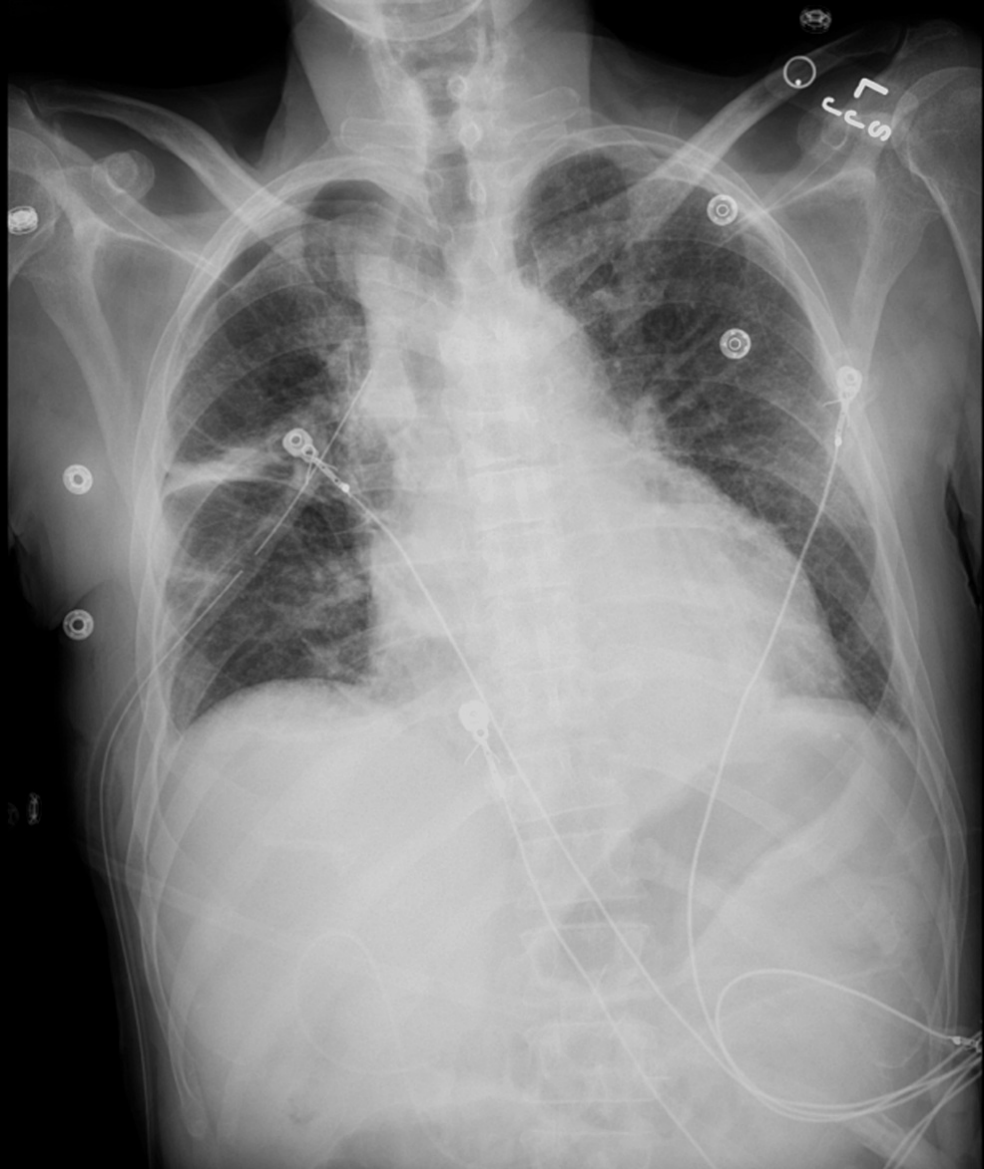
Chest X-ray post right-sided chest tube insertion
